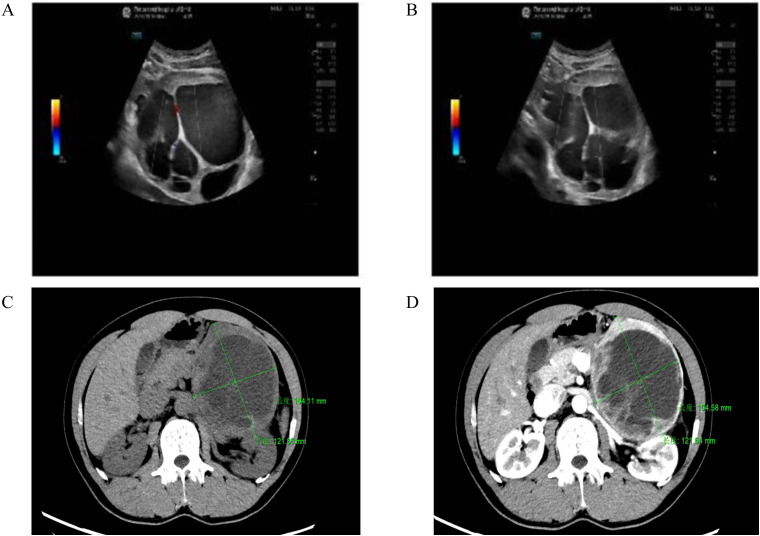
Preoperative imaging. (A, B) Color Doppler ultrasonography revealed a predominantly cystic mixed-echo mass located in the pancreatic tail region. (C, D) Contrast-enhanced CT demonstrated a large heterogeneous cystic-solid mass with mild enhancement of the cyst wall, septa, and solid components.
